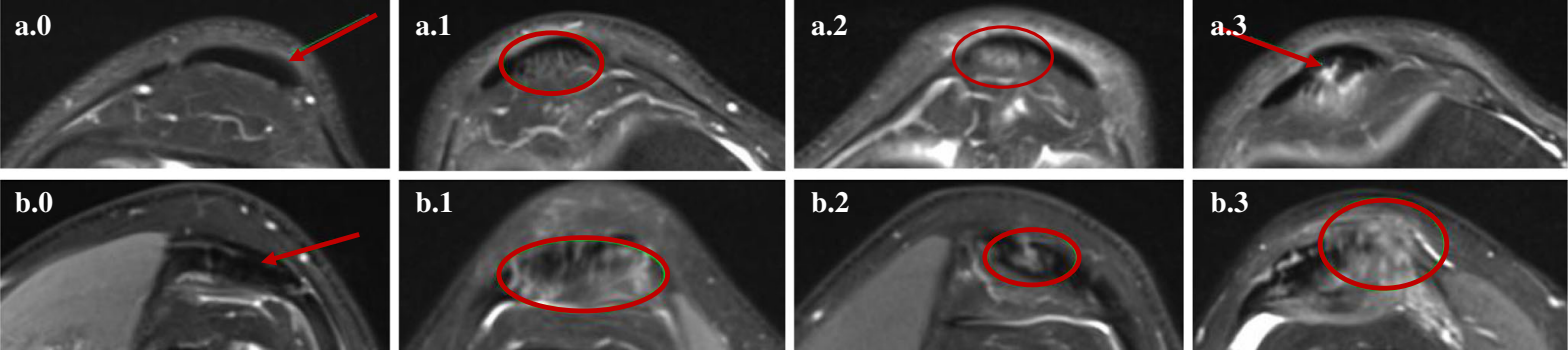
Axial proton-density fat-saturated MR images of (a) patellar tendons (a.0, grade 0; in the left knee of a 40-year-old man; a.1, grade 1; in the right knee of a 62-year-old man; a.2, grade 2; in the left knee of a 56-year-old man; a.3, grade 3; in the right knee of a 44-year-old man) and (b) quadriceps tendons (b.0, grade 0; left knee of a 40-year-old man; b.1, grade 1; in the right knee of a 40-year-old woman; b.2, grade 2; in the left knee of a 44-year-old man; b.3, grade 3; in the right knee of a 48-year-old man). The tendons are indicated by red arrows or circles; grade 0: normal tendon appearances; grade 1: increased signal intensity in less than 25% of the axial cross-sectional tendon width; grade 2: increased high-signal intensity in 25 to 50% of the axial cross-sectional tendon width; grade 3: increased high-signal intensity occupying more than 50% of the axial cross-sectional tendon width
